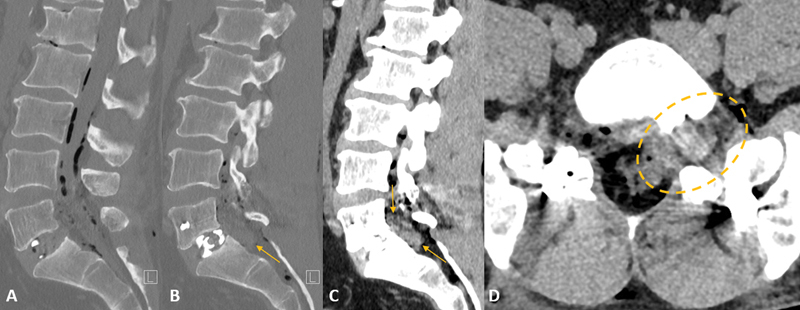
Postoperative lumbar spine computed tomography after 1-day. (
